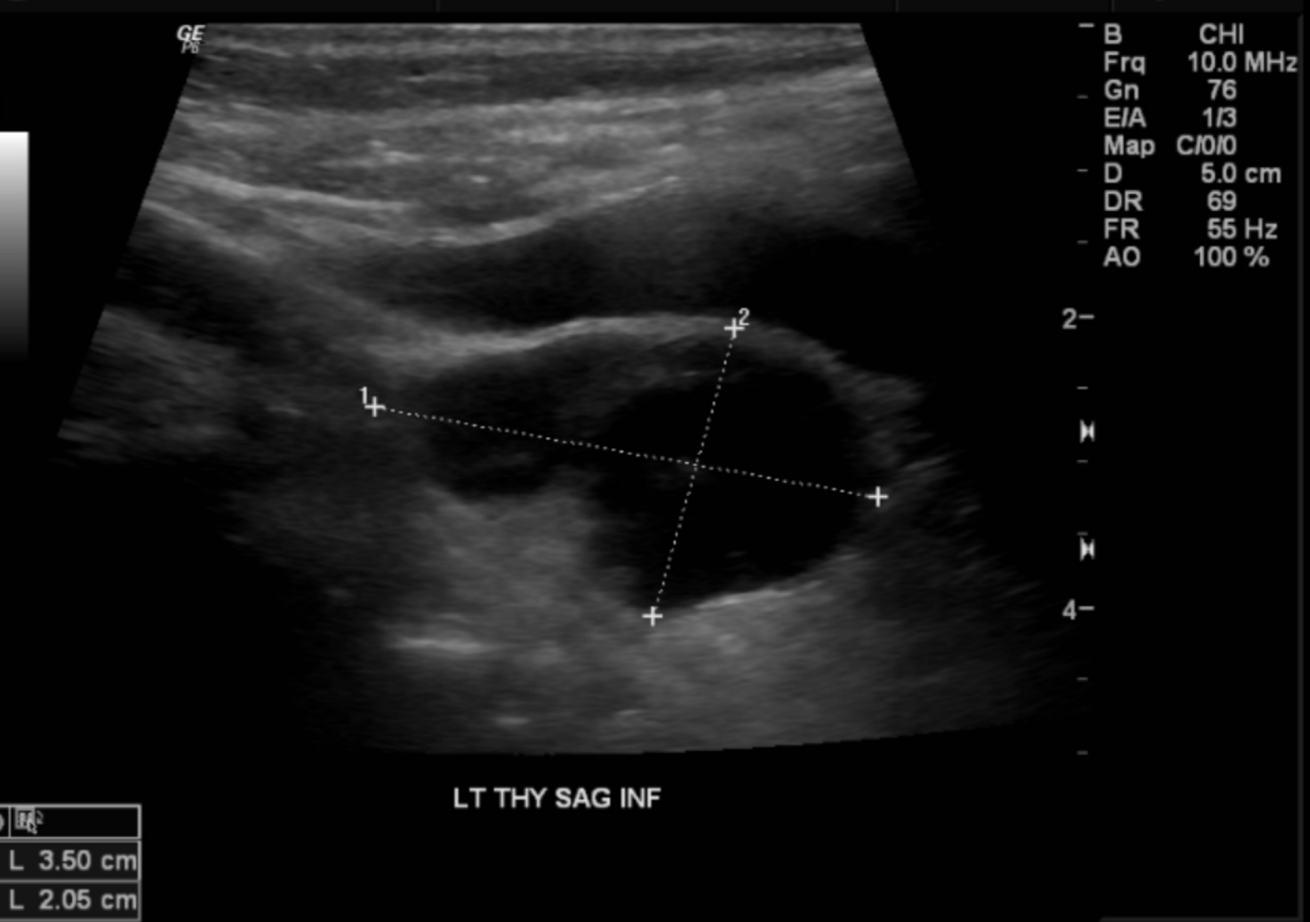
Thyroid and parathyroid ultrasound revealing a predominantly cystic appearing mass with septations and mild irregularity measuring roughly 3.5 x 2.1 x 2.0 cm
